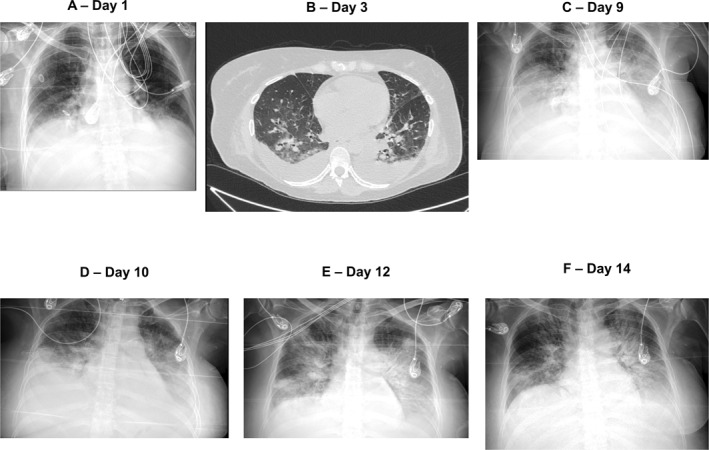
X‐rays/computed tomography scans of the patient's chest following brexucabtagene autoleucel administration on (A) day 1, (B) day 3, (C) day 9, (D) day 10, (E) day 12, and (F) day 14.
